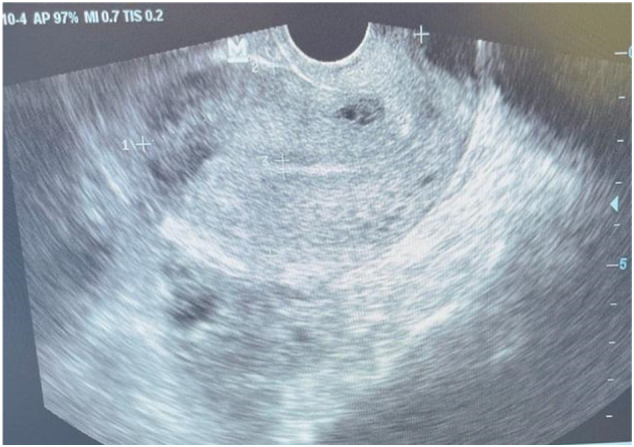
Ultrasound image of uterine vacuity.
